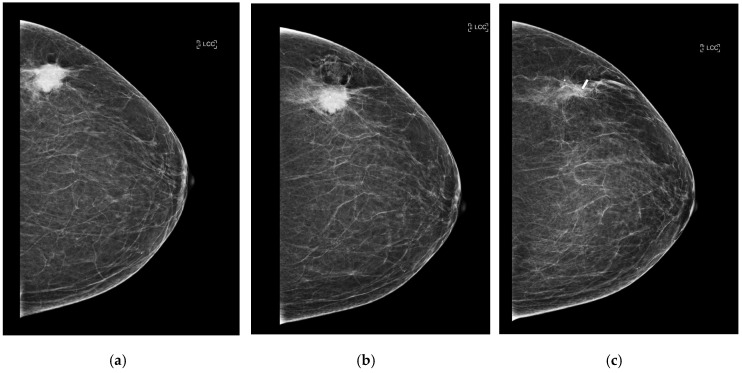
(a) Craniocaudal (CC) view of the left breast of predominately fat density (BI-RADS A). There is a 27 mm malignant-appearing mass in the upper portion of the left breast. (b) CC post-biopsy mammogram shows the marker clip within the center of the lesion. Following ultrasound-guided core needle biopsy and accurate clip placement, biopsy results yielded invasive ductal carcinoma (grade 3). The patient underwent neo-adjuvant chemotherapy followed by radiofrequency RFID tag localization. (c) CC view of the left breast. The known mass within the upper outer quadrant has reduced in size following neo-adjuvant chemotherapy and measures 19 mm (from 27 mm initially). The post-localization mammogram shows successful RFID tag placement (at the lateral aspect of the lesion).
